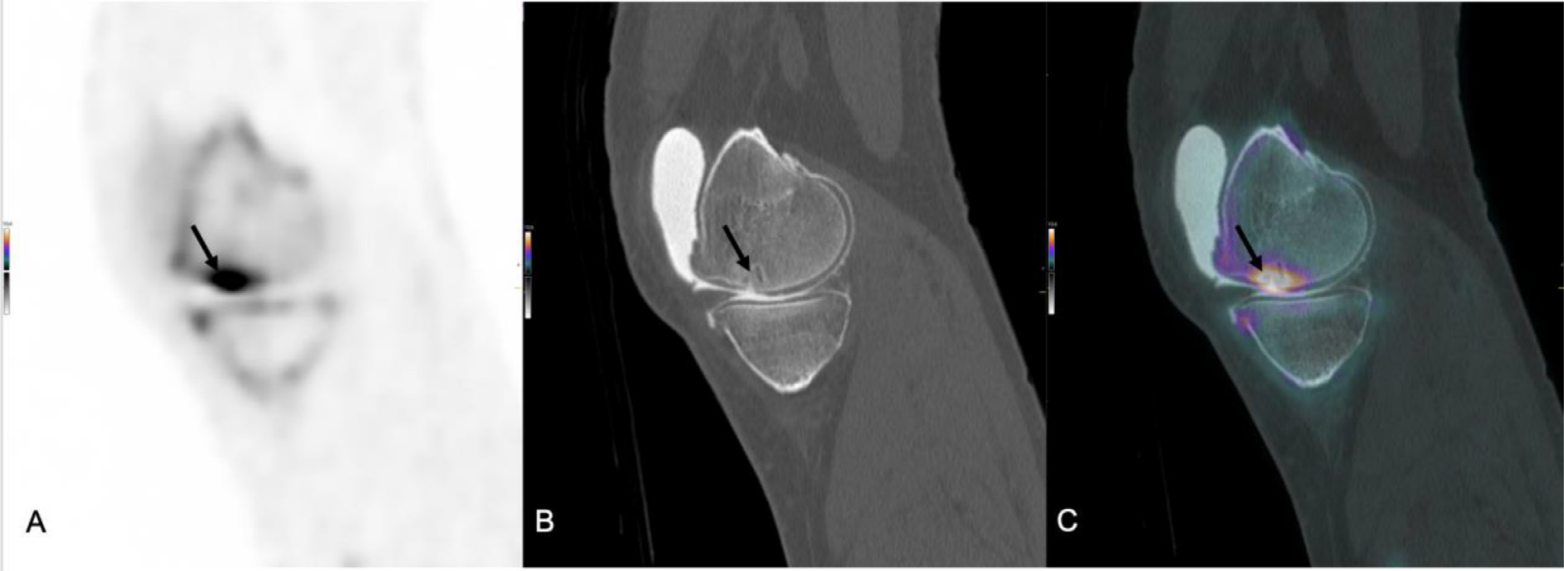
Sagittal images of a sodium fluoride-18 PET/CT (Na[^18^F]F-PET/CT) scan with intra-articular contrast (A, PET; B, CT; C PET/CT fusion) with an intense Na^18^F focus (16 × 15 mm) with Grade 4 cartilage defect, mild sclerosis, and a small subchondral cyst (arrow). Small spike-like indentations filled with contrast, consistent with degenerative cartilage, are present elsewhere in the retropatellar cartilage.
